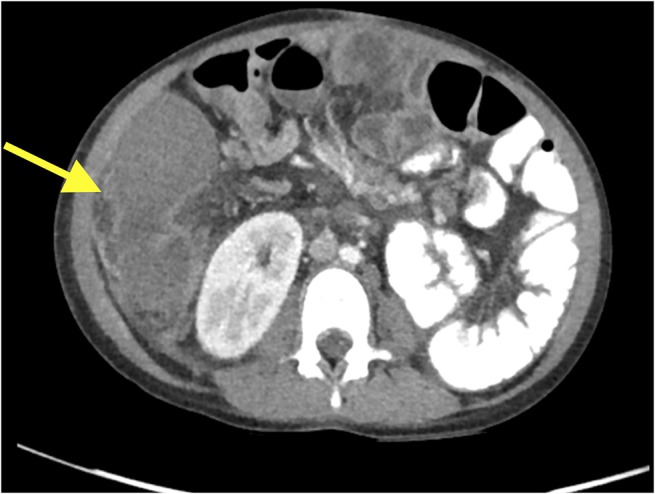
Axial CT scan demonstrating a right intra-abdominal rim enhancing lesion.
